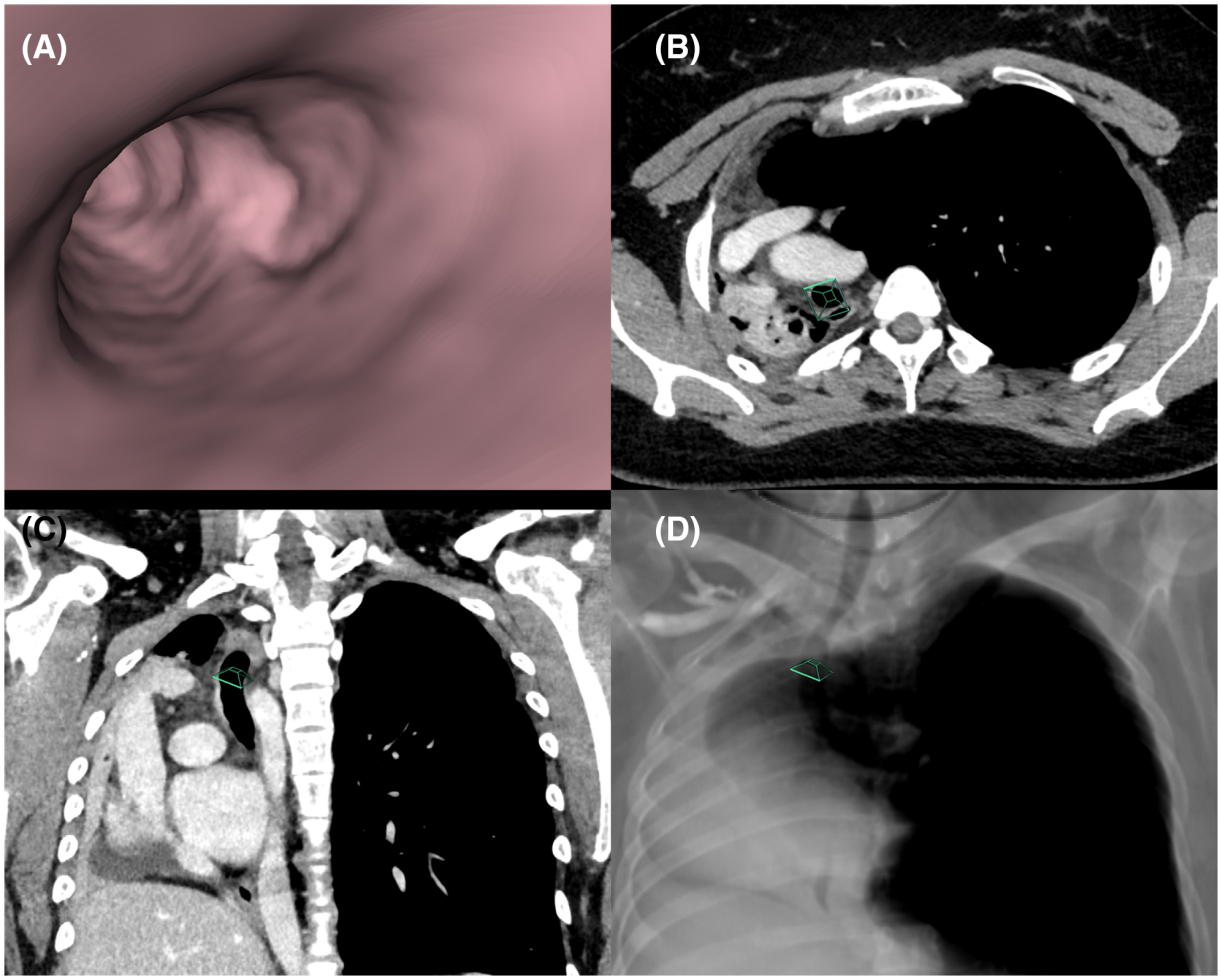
Chest computed tomography (CT) images. (A) Identification of the right main bronchial obstruction site using virtual bronchoscopy. (B) Axial image of the obstruction site. (C) Coronal image of the obstruction site. (D) RaySum (Ray Summation) constructed from CT images.
